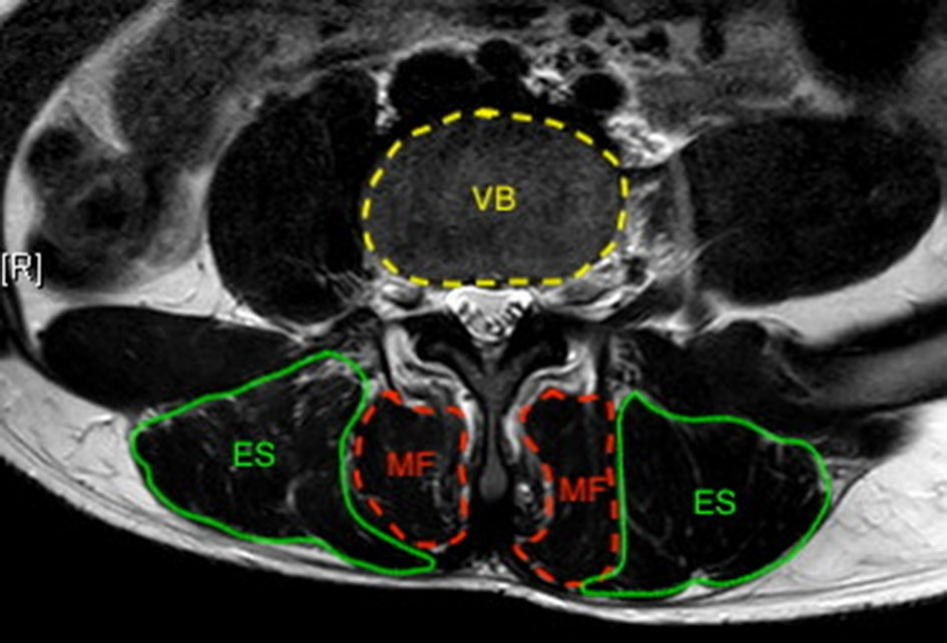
Measurement of cross-sectional area (CSA) of erector spinae (ES) and multifidus (MF) and L4 vertebral body using magnetic resonance imaging. CSA of muscle was calculated as the mean value of bilateral CSA. Muscularity = CSA of muscle − vertebral body ratio.
